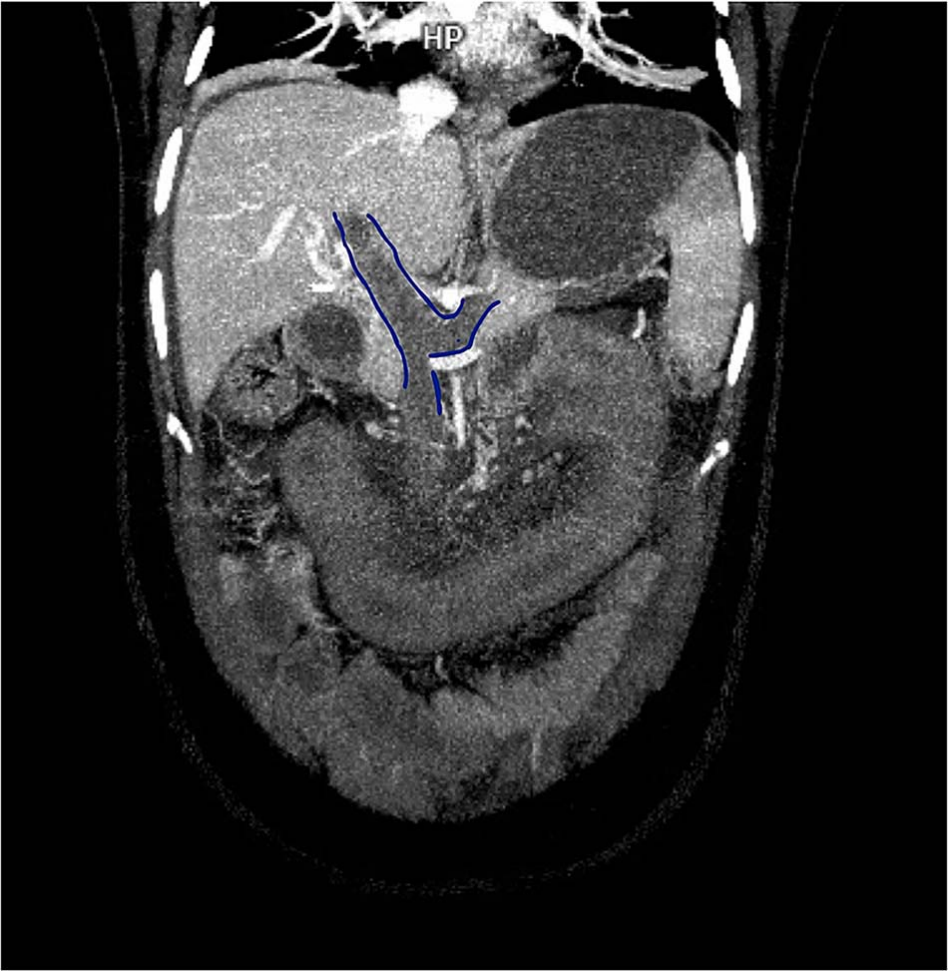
Contrast-enhanced CT in venous phase showing thrombosis of the portal, splenic, and superior mesenteric veins with proximal dilatation and thickening of the jejunal wall.
